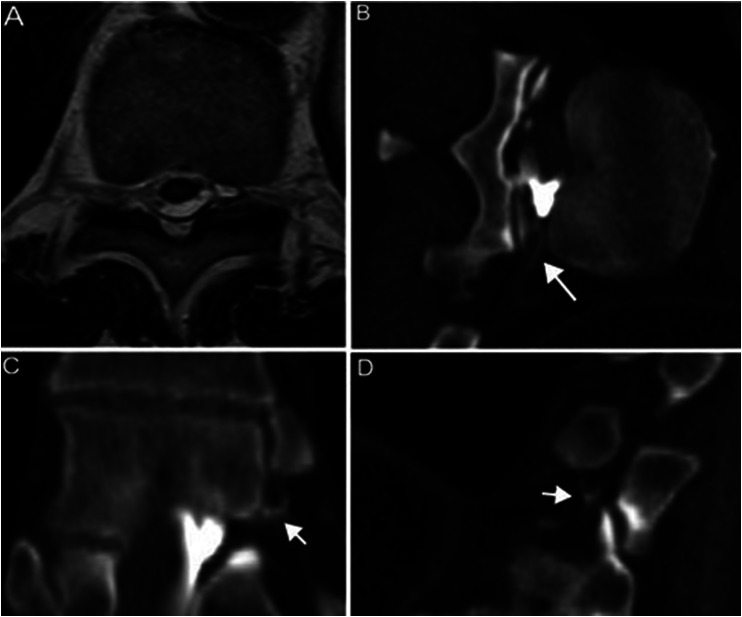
Heavily T2-weighted axial magnetic resonance imaging (MRI) demonstrating a small fluid collection in the medial portion of the left T10–T11 neural foramen (A), either compatible with a T10 nerve root dural ectasia or with a localized cerebrospinal fluid (CSF) leak. Left lateral decubitus computed tomography (CT) myelogram demonstrated a CSF-venous fistula at that level with drainage in the left T10 intersegmental vein (B, long arrow) and in multiple small periforaminal veins (C, D, short arrows).
